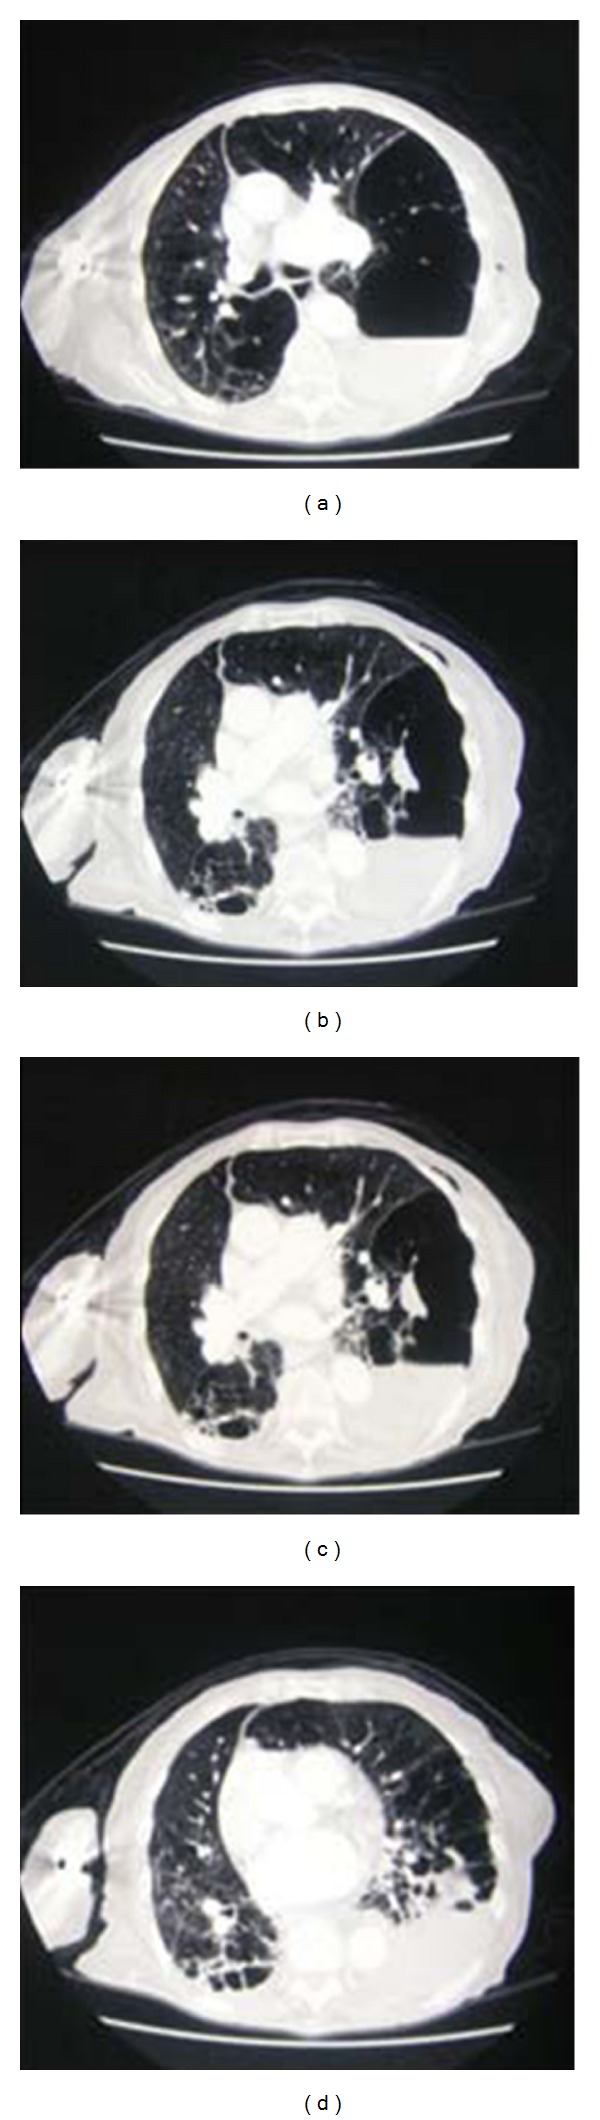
A CT scan on the same patient as in Figure 11 shows extensive bullous emphysema. The punctured bulla contains an air/fluid.
